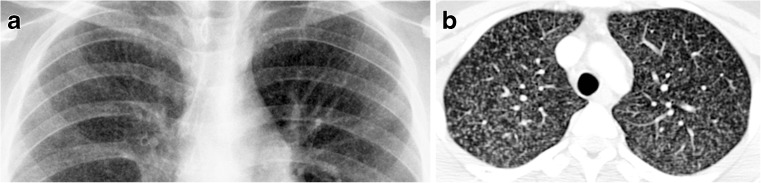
Miliary tuberculosis in a 14-year-old boy. a, b Focused anteroposterior chest radiograph (a) and axial contrast-enhanced CT (b) of the upper lobes show innumerable 2-mm or smaller discrete nodules
